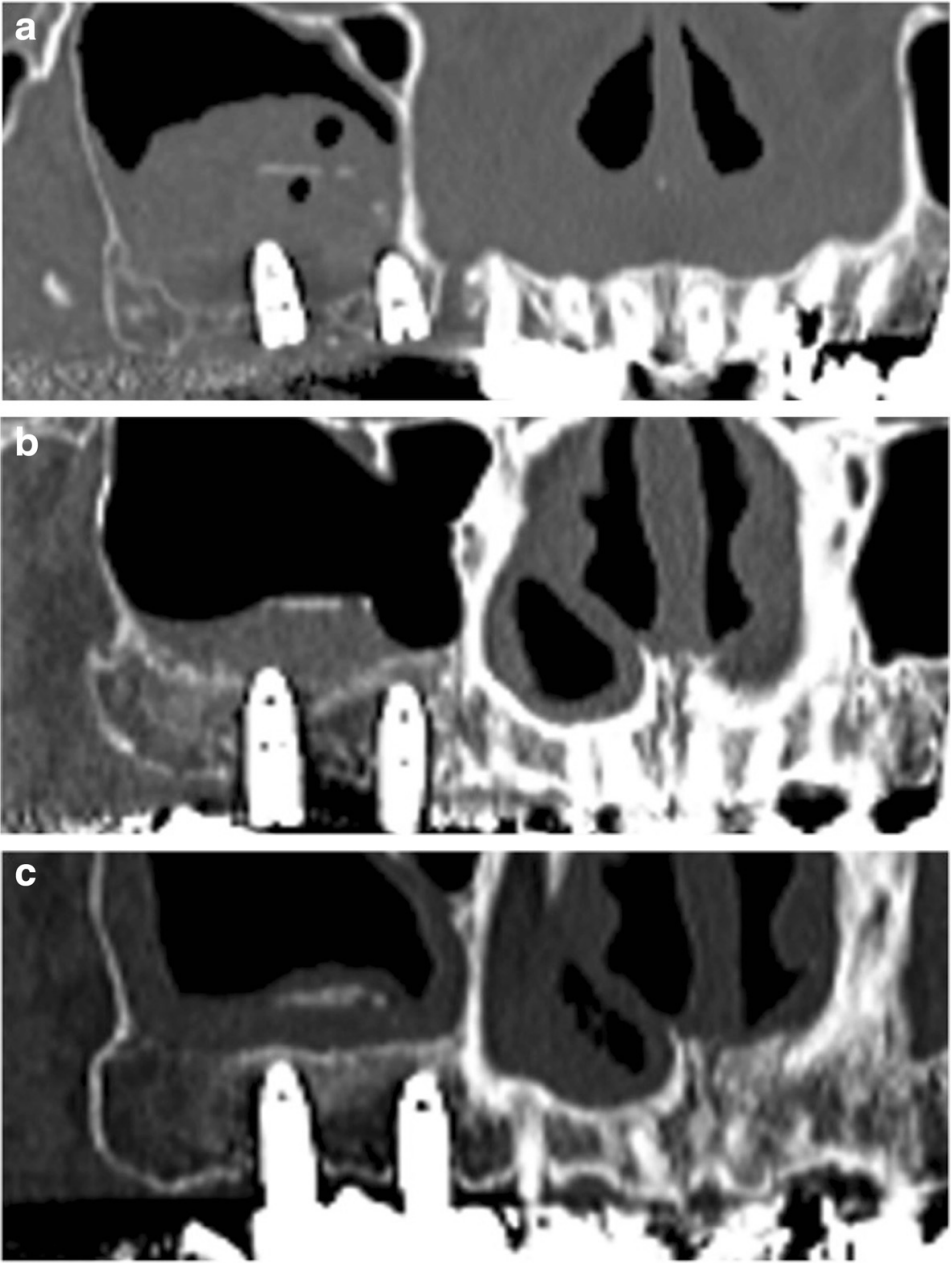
Radiographic findings on panoramic computed tomography after nongrafted sinus lift with simultaneous implant placement. a Immediately. b At 6 months. c At 42 months
